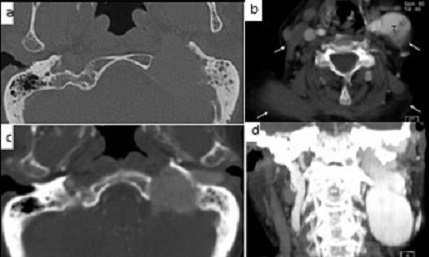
A) computerized tomography, axial image demonstrates large extensive bone erosion and destruction of temporal and jugular bulb; B) post contrast CT angiography (CTA) axial image revealed a solid, well defined mass that seemed to be a highly vascularized tumor: notable atrophy of the trapezius and sternocleidomastoid muscle (white arrows), which indicate invasion of the left cranial nerve XI; C) CTA showed an important enhancement with extension on periauricular region; D) coronal CTA thick image demonstrated the extension in height of the tumor
